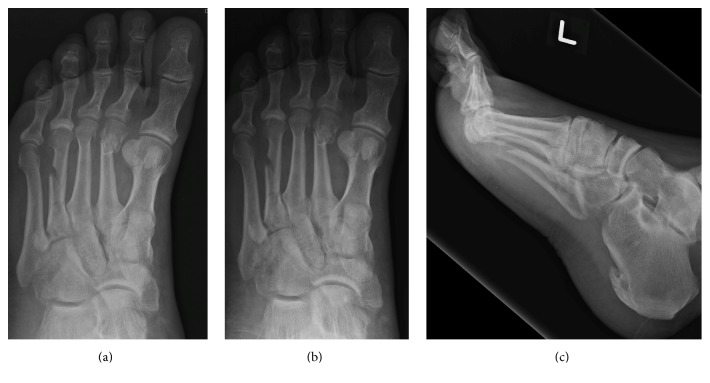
Dorsoplantar (a), oblique (b), and lateral (c) radiographs of the left foot at initial presentation.
